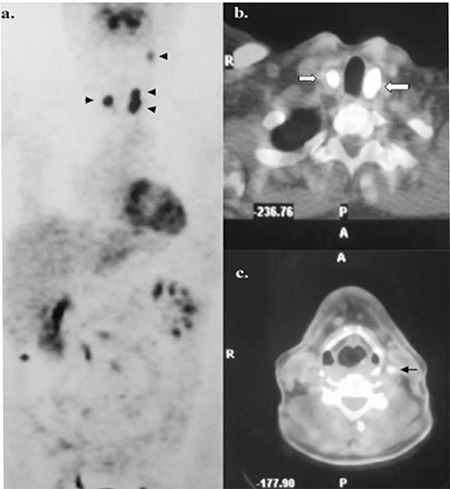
Preoperative 18F-FDG whole-body PET (a) and axial PET-CTfusion (b, c) images show pathological uptakes (arrowsheads) in boththyroid beds (white arrows) and a left jugular lymph node (black arrow).
